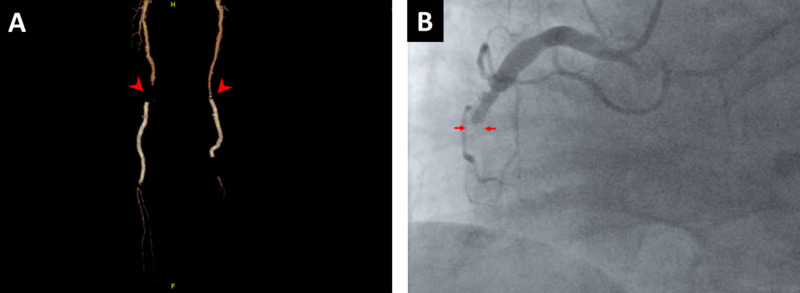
3D-Run off reconstruction from CT lower extremity angiogram (A) and selective left heart catheterization (B). A: Bilateral popliteal stents are shown, with bilateral proximal filling defect (red arrowheads) and poor distal reconstitution, indicating stenosis. B: Large ectatic RCA, with abrupt mid-RCA filling defect, diagnostic for 100% in stent thrombosis (red arrows). RCA, right coronary artery
